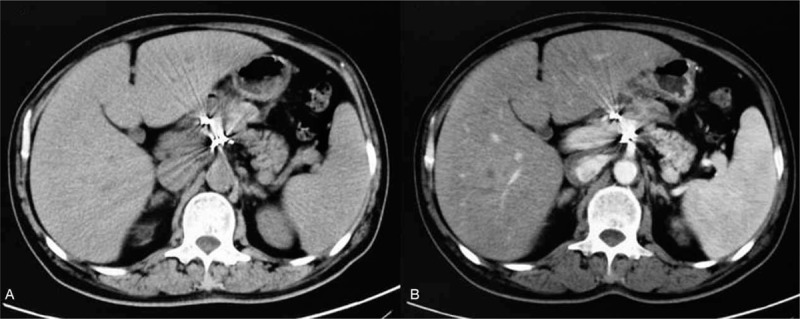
Enhanced CT scans 2 mo after intervention. (A) Non-contrast-enhanced phase. (B) Artery phase. The hematoma significantly diminishes in the imaging. CT = computed tomography.
